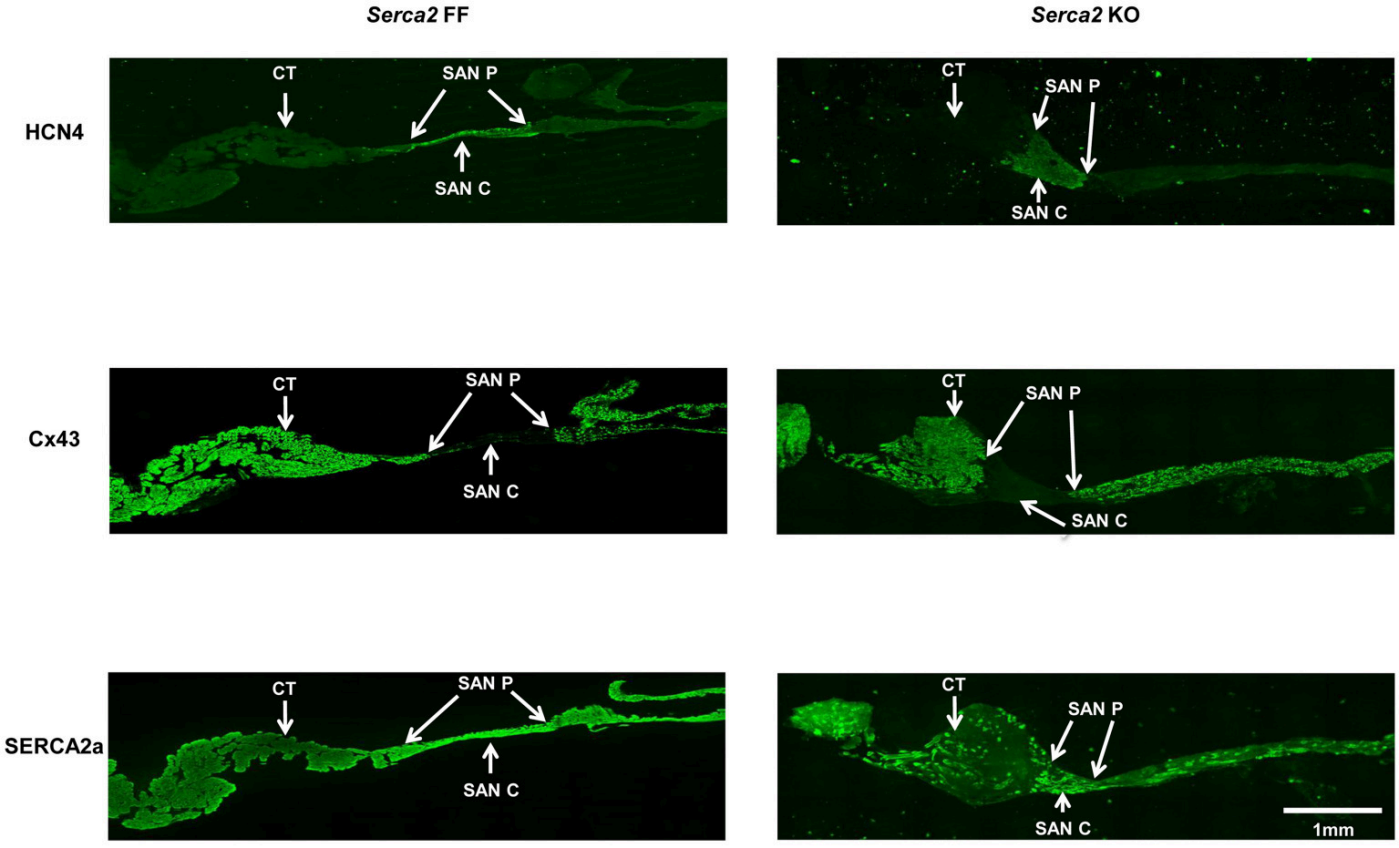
Immunolabelling of HCN4, Cx43, and SERCA2 in right atrial sections from *Serca2* FF and *Serca2* KO hearts. Top row, right atrial sections from *Serca2* FF (left) and *Serca2* KO (right) hearts showing HCN4 labeling (green signal) in the sinus node periphery (SAN P) and center (SAN C). Middle row, connexin 43 (Cx43) labeling (green signal) in the right atrium. Bottom row, right atrial sections showing SERCA2a labeling (green signal) throughout the tissue section in *Serca2* FF (left) and a mosaic pattern of SERCA2a labeling in *Serca2* KO (right). *Abbreviations*: CT, *crista terminalis*; SAN P, sinus node periphery; SAN C, sinus node center.
